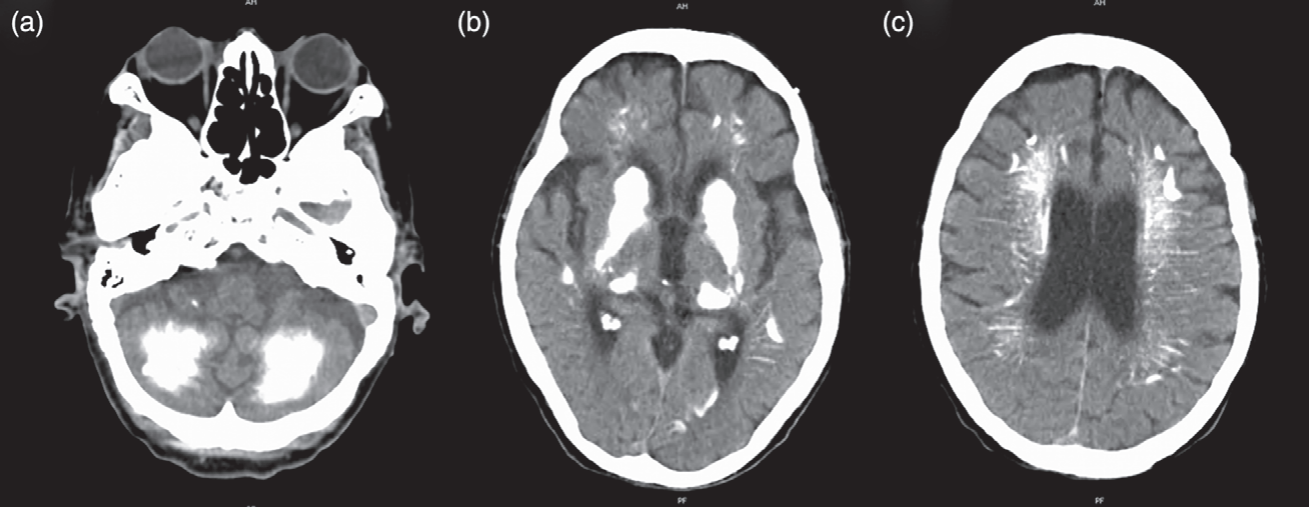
Computed tomographic scan of the patient's brain. CT scans show extensive, bilateral calcification of dentate nuclei and cerebellar white matter (a), basal ganglia (b) and centrum semiovale and periventricular white matter (c).
